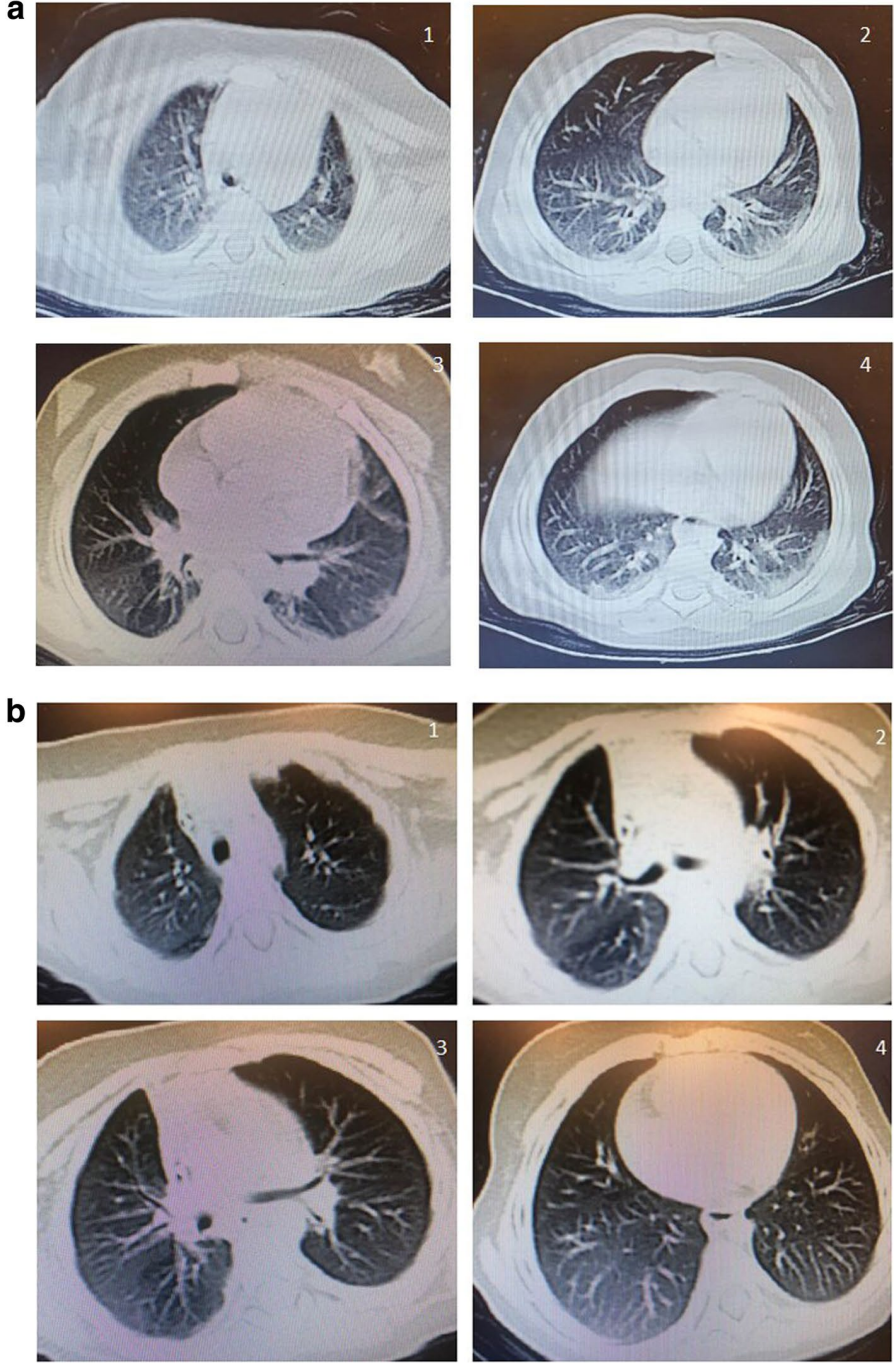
a Case 2 initial serial CT cuts showing bilateral multiple pulmonary asymmetric variably defined multilobar areas of ground-glass opacities predominantly peripheral with sub-pleural posterior consolidations with related accentuated vascularity and air space pattern involving all both lung lobes (CO-RADS 4). b Follow-up case 1 serial CT cuts showing significant regressive course with marked resolution, but still noted ground glass veiling and bronchial thickening denoting post inflammatory small airway disease sequels
